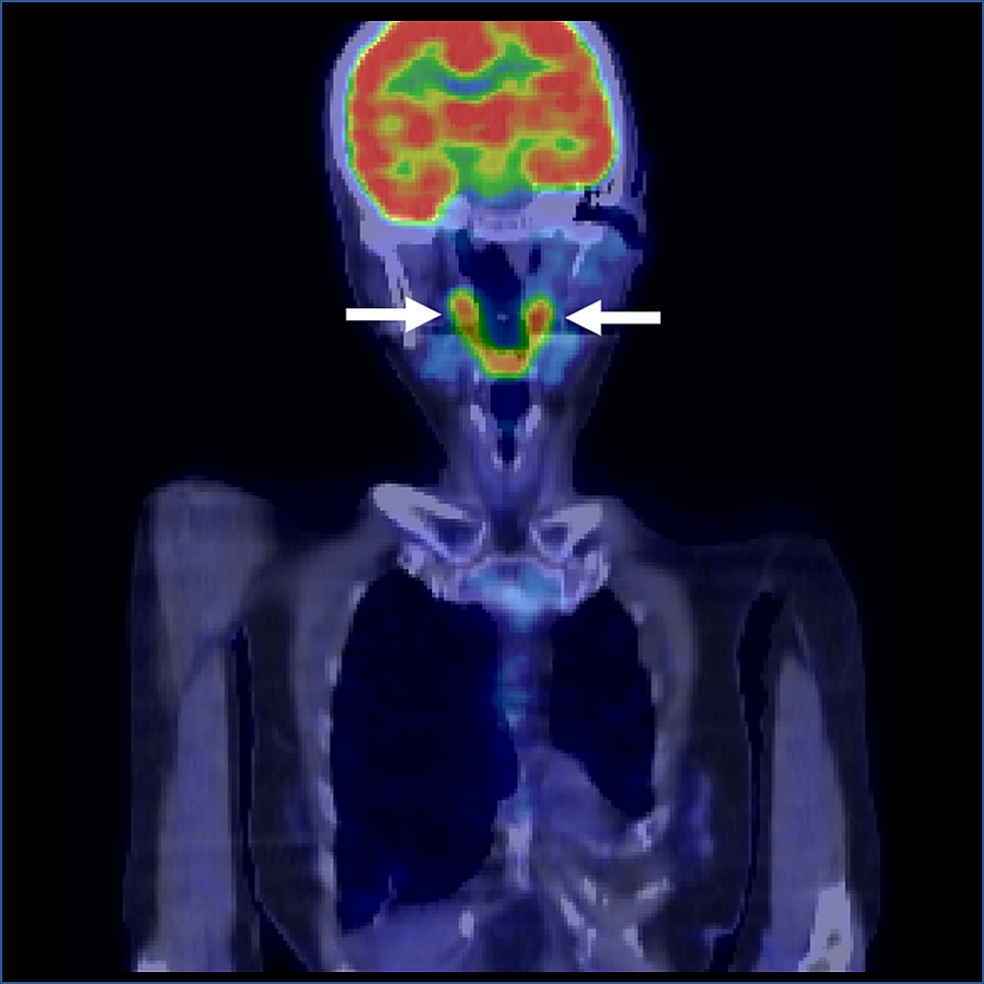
FDG-PET-CT. FDG-PET-CT revealed accumulations in the carotid arteries and pulmonary arteries bilaterally FDG-PET-CT, 18F-fluorodeoxyglucose-positron emission tomography-computed tomography
